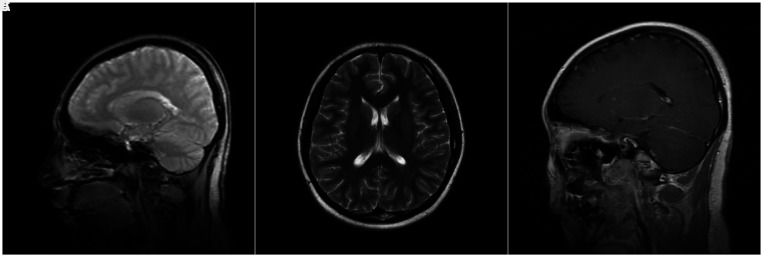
(A, B, and C) Magnetic resonance imaging of the head (August 2018) reveals that cystic granulomas almost disappeared after four courses of treatment.
